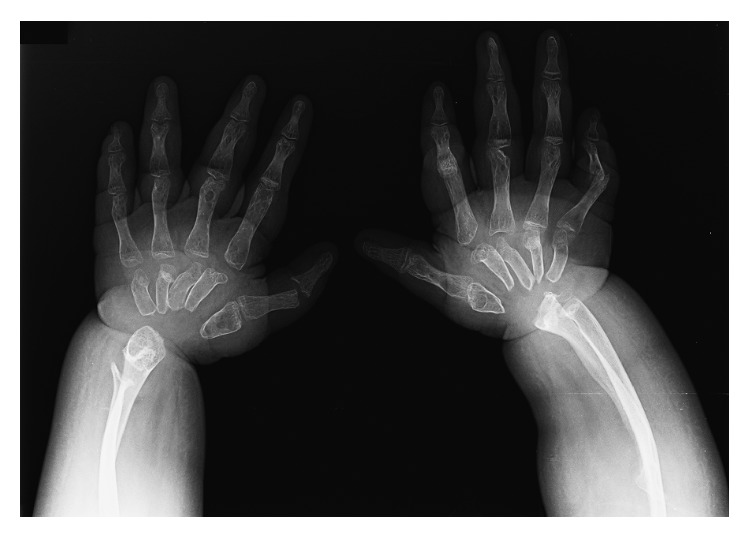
Plain hands radiograph demonstrating the absence of carpal bones and osteolysis of the proximal end of metacarpal bones as well as the resorbed distal end of ulna and radius. Severe cortical thinning of metacarpal and phalangeal bones as well as bowing of ulna and radial bones was observed.
